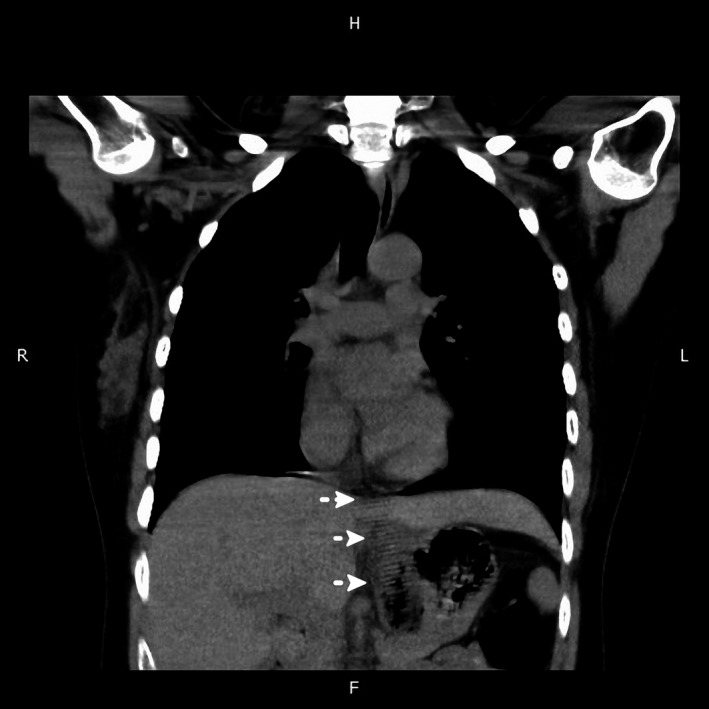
Coronal reconstructed noncontrast abdominal computed tomography revealing a comb‐shaped opacity within the stomach (white arrow).
